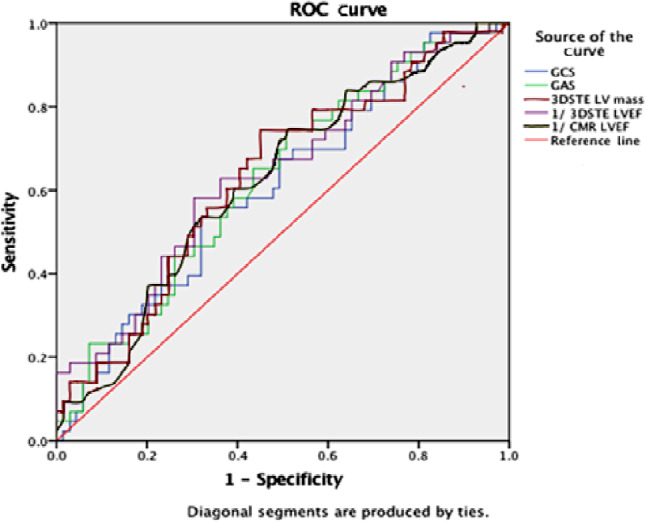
Receiver-operating characteristic (*ROC*) curve analysis for the prediction of cardiac events. Left ventricular ejection fraction (*LVEF*) is the most sensitive parameter with a sensitivity of 61% (*3DSTE* three-dimensional speckle tracking echocardiography, *CMR* cardiac magnetic resonance, *GAS* global area strain, *GCS* global circumferential strain)
